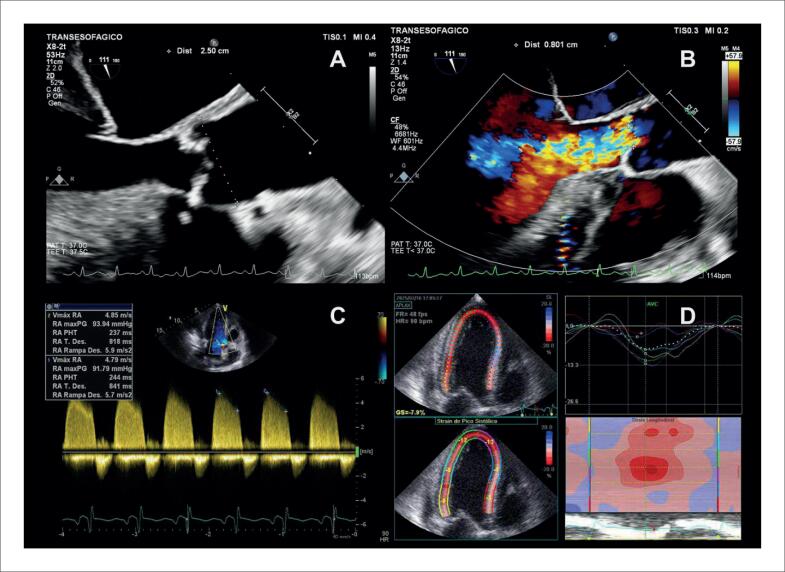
Imagens ecocardiográficas de paciente com diagnóstico de lúpus eritematoso sistêmico com valvulite aórtica e insuficiência aórtica de grau importante (A, B e C), além de miocardite evidenciada pela redução do SLG do ventrículo esquerdo ao corte três câmaras (D) (SLG = −7,9%) (D). Em A, ao ecocardiograma transesofágico, evidencia espessamento moderado e retração discreta da abertura da valva aórtica avaliada em eixo longo, associada a insuficiência de grau importante ao Doppler colorido (B). Em C, curva espectral ao Doppler contínuo com jato denso associado ao volume regurgitante significativo e redução do tempo de meia pressão (PHT = 237 ms). ETE: ecocardiograma transesofágico; PHT: tempo de meia-pressão (pressure half-time); SLG: strain longitudinal global.
